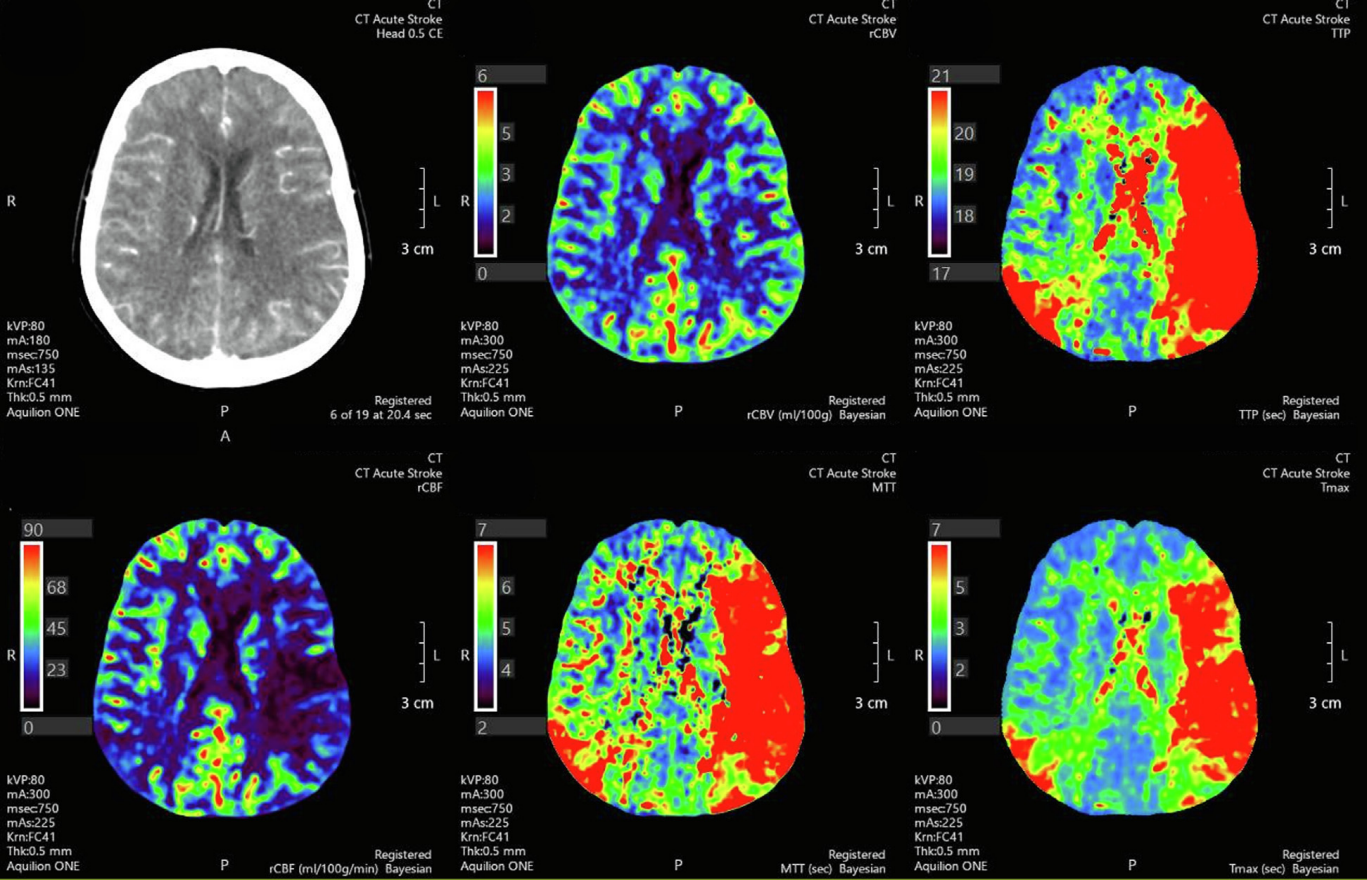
Brain Computed Tomography Computed tomography showing a large multiterritorial ischemic stroke.
